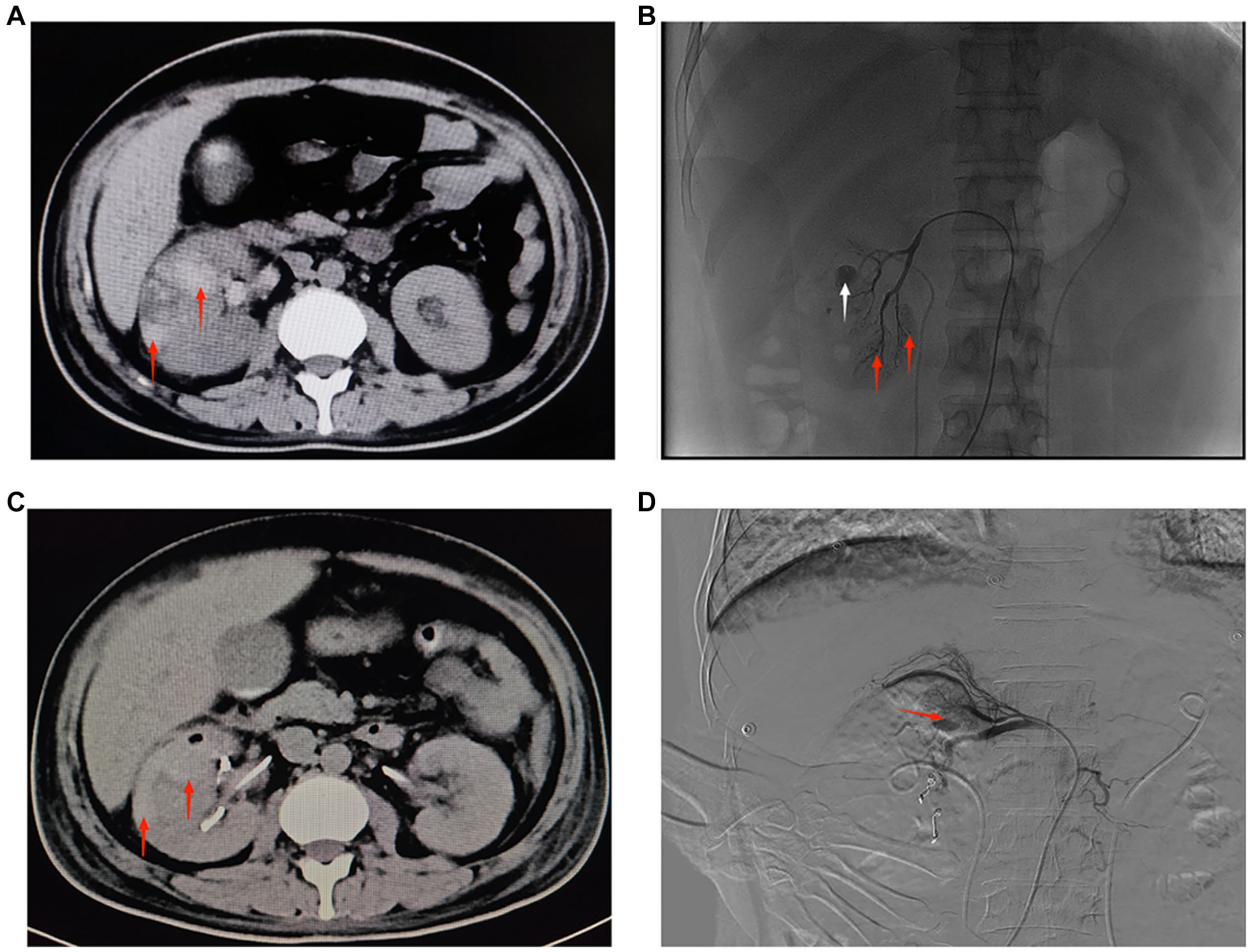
(A) CT of both kidneys. The right kidney is increased in size, heterogeneous in density, with multiple flaky hyperdense shadows, and clear perirenal space in both kidneys. The red arrow shows a hematoma in the lower pole of the right kidney (September 19, 2022, day 16 of admission). (B) Angiography of the right renal artery. Red arrows show active extravasation of multiple subsegmental arteries supplying the lower pole. White arrow indicates pseudoaneurysm formation (September 19, 2022, day 16 of admission). (C) CT of both kidneys. The right kidney is increased in size, heterogeneous in density, with multiple patchy hyperdense shadows, and clear perirenal space in both kidneys. The red arrow shows a hematoma in the upper pole of the right kidney (September 22, 2022, day 19 of admission). (D) Digital subtraction angiography of the right renal artery. Red arrow indicates active extravasation of the subsegmental artery supplying the superior pole (September 22, 2022, day 19 of admission).
